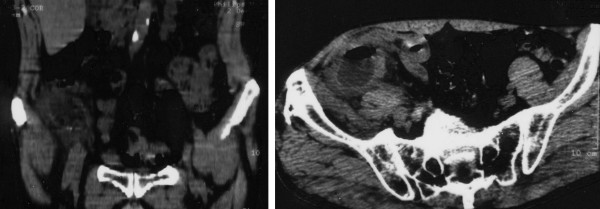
Abdomino-pelvic CT scan reveals a 6 × 8 cm right iliac fossa abscess with a remarkable wall thickening of the adjacent cecum.
